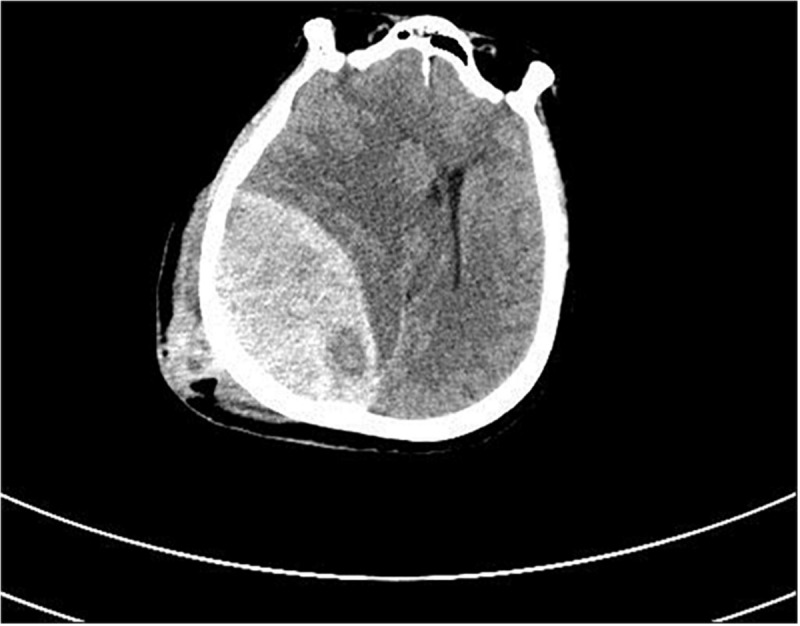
The CT examination of one patient with cerebral contusion and laceration with intracerebral hematoma.
